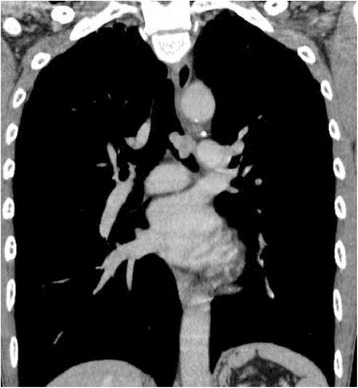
CT with multiplanar reconstruction revealed a polypoid tumor originating at the lateral and distal part of the trachea and protruding into the airway
